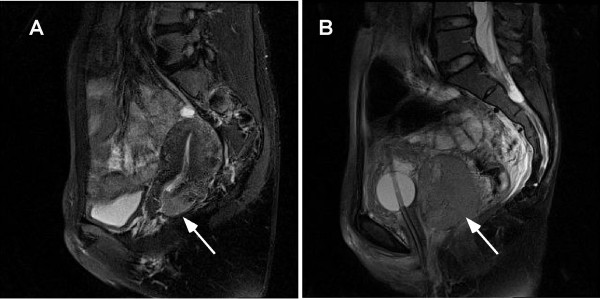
Magnetic resonance imaging of primary and recurrent cervical mass. A. The arrow indicated a 2.7*2.2*3.4 cm lesion in the posterior cervix, accompanied by disappearance of the posterior fornix. B. The arrow indicated a 68*63*58 mm mass in the vaginal cuff.
